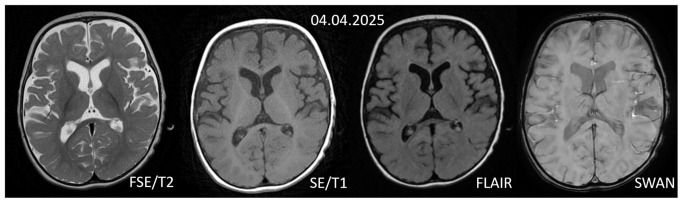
Brain MRI figures performed at the age of 8 months (4 April 2025): FSE/T1, SE/T2, FLAIR and SWAN sequence images from the examination of 4 April 2025 showing normal signal of brain structures, including both thalami and internal capsules. The examination was performed with a GEMS Artist 1.5T device. Abbreviations: MRI—Magnetic Resonance Imaging, FSE/T1—Fast Spin Echo/T1-Weighted, SE/T2—Spin Echo/T2-Weighted, FLAIR—Fluid-Attenuated Inversion Recovery, SWAN—Susceptibility-Weighted Angiography.
